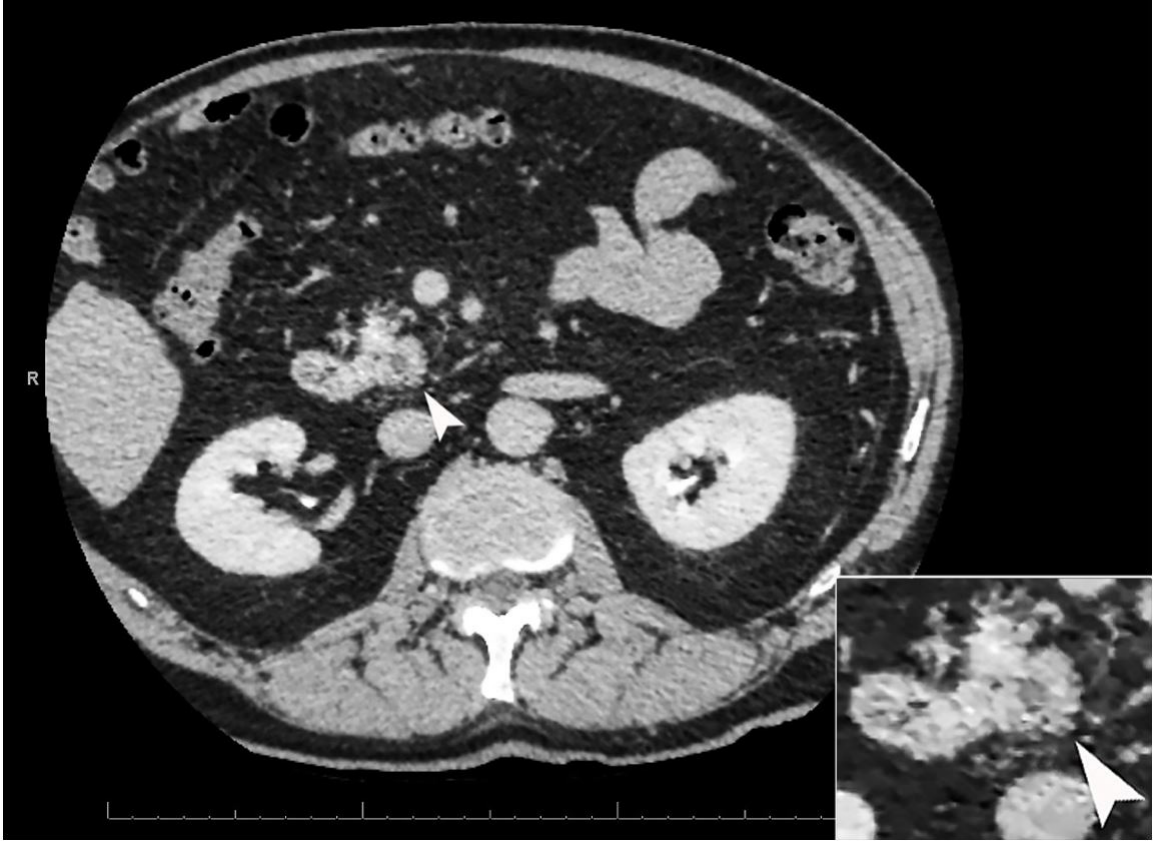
Abdominal axial computed tomography (CT) scan shows a 0.7 cm hypodense mass in the pancreas (arrow).
